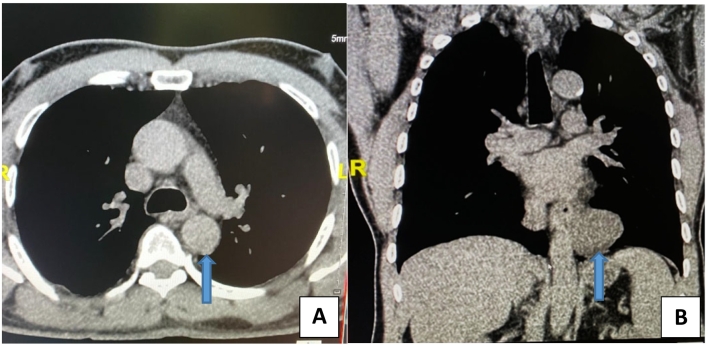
A rounded cystic mass (arrow) of the posterior mediastinum, axial (A) and coronal (B) chest CT scan.
